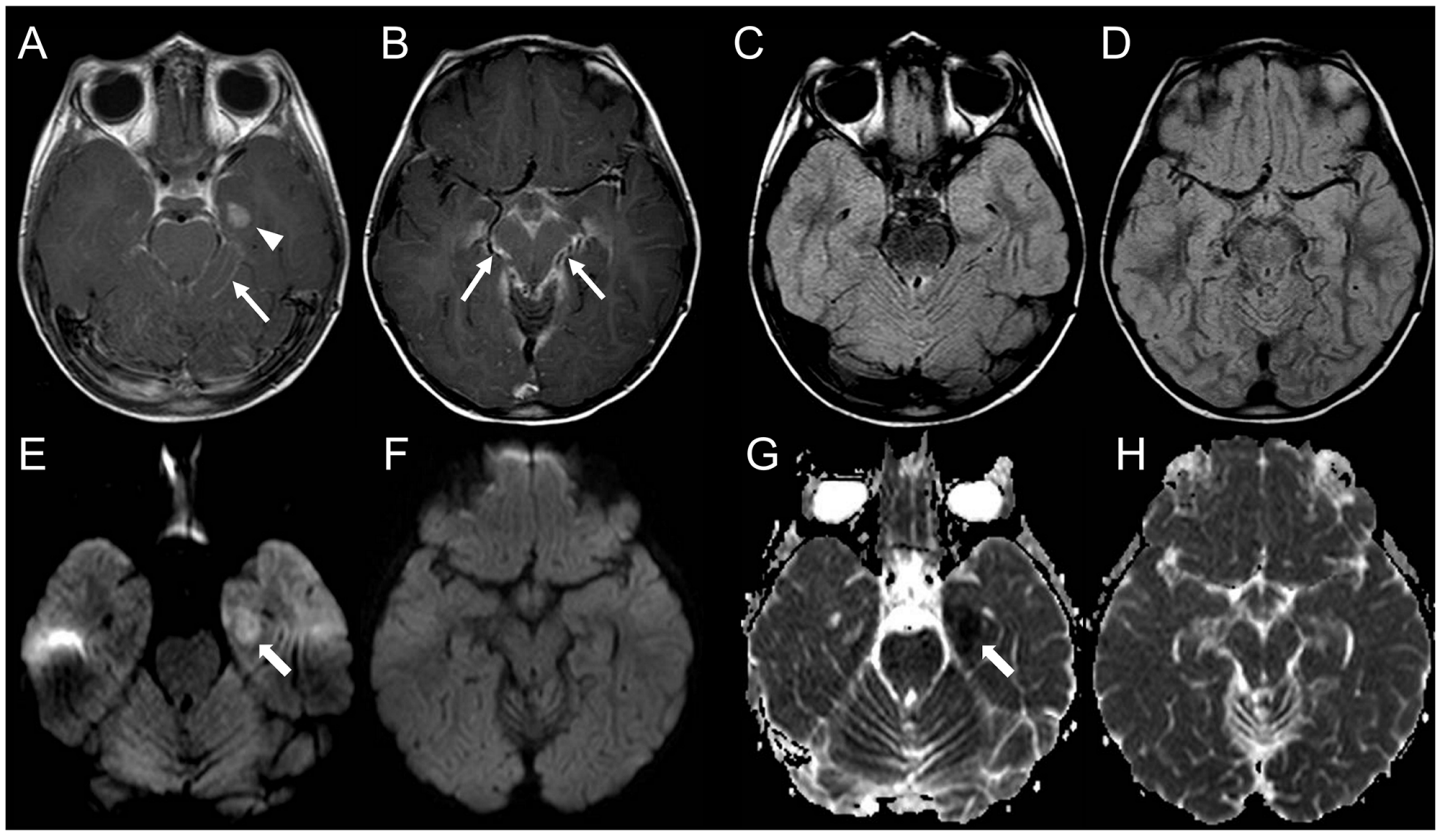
Brain MRI findings in a 7-year-old girl with AT/RT relapse and linear leptomeningeal involvement not visible on DWI Post-contrast T1-weighted images show a distant focal nodular contrast enhancing relapsing lesion in the left temporal lobe (arrowhead, A); there is also diffuse linear leptomeningeal enhancement along cerebellar folia and in the basal cistern (long thin arrows, A, B). FLAIR images do not reveal evident lesions (C, D). DWI and corresponding ADC maps clearly show the nodular lesion with decreased diffusivity in the left temporal lobe (thick arrows, E, G) but are unable to demonstrate linear leptomeningeal involvement (F, H).
